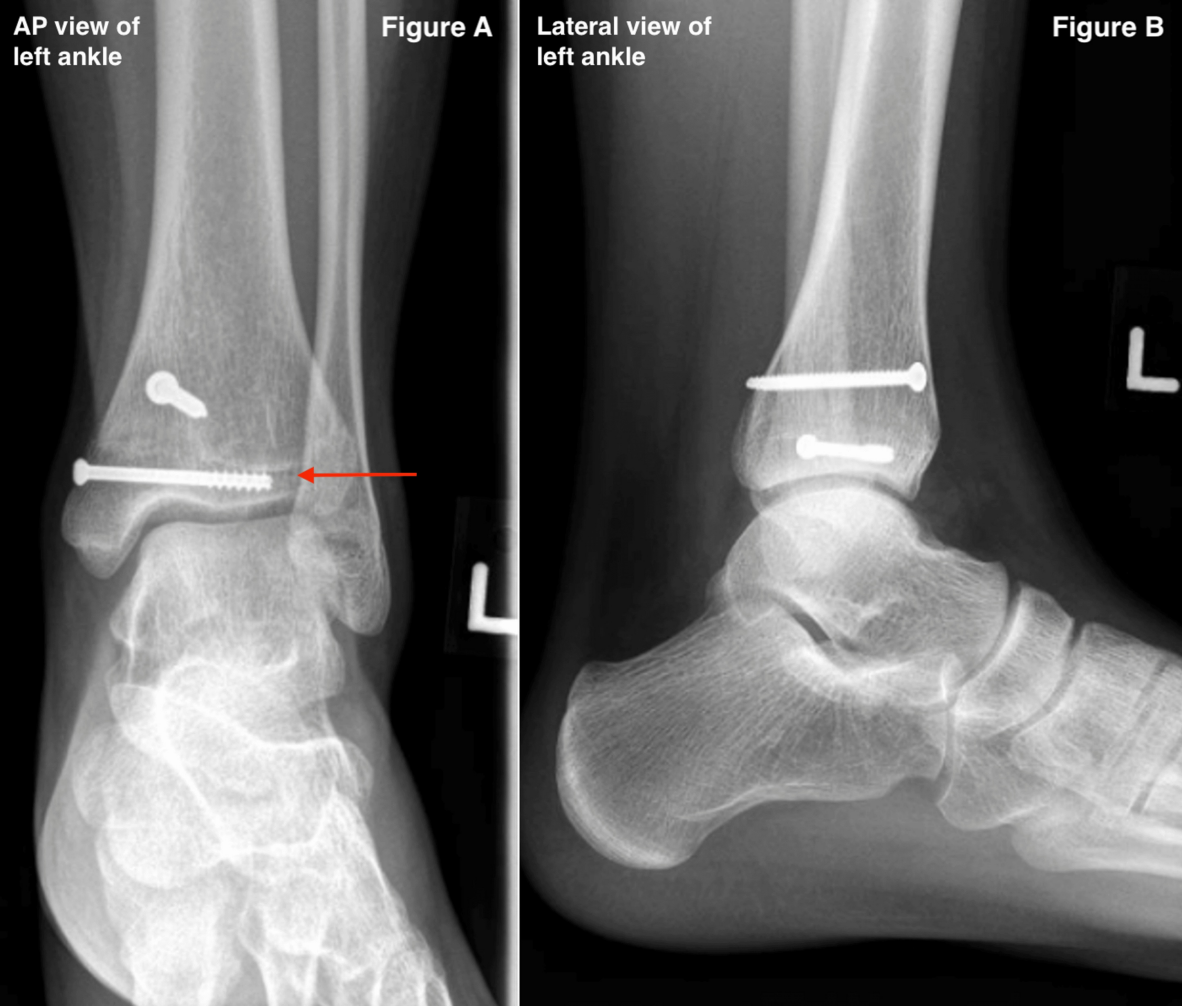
Postoperative radiographs showing healed fracture, with anteroposterior (A, left) and lateral views (B, right) of the left ankle. Arrow: Fracture fragments are held together by 4.0 mm short-thread cannulated screws for healing to occur. AP: anteroposterior.
